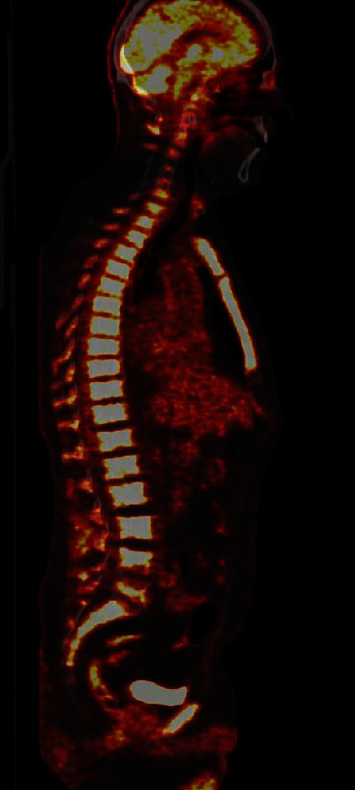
[F^18^]FDG-PET-CT showing intense uptake in the central bone marrow.
